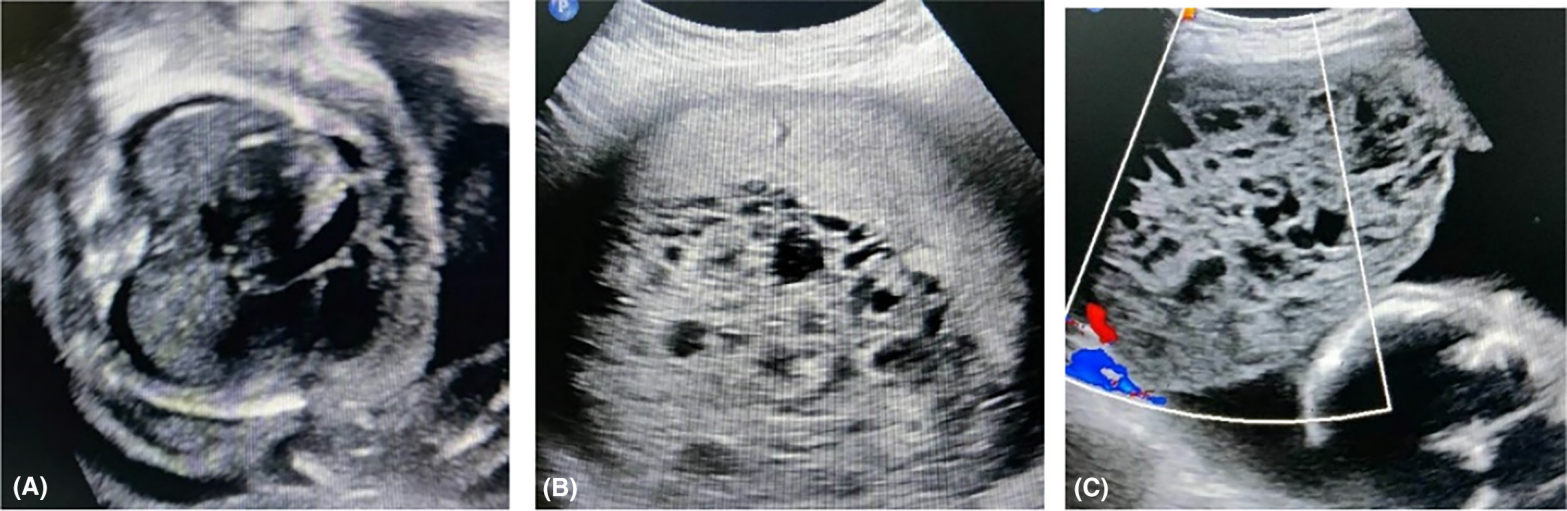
Ultrasound scans indicative of partial mole with hydrops fetalis in the dead fetus. Hypoechoic fluid (A) is seen in the dependent aspect of the bilateral pleural cavity and pericardial cavity. B and C show the part of the placental tissue with multiple cystic anechoic areas.
